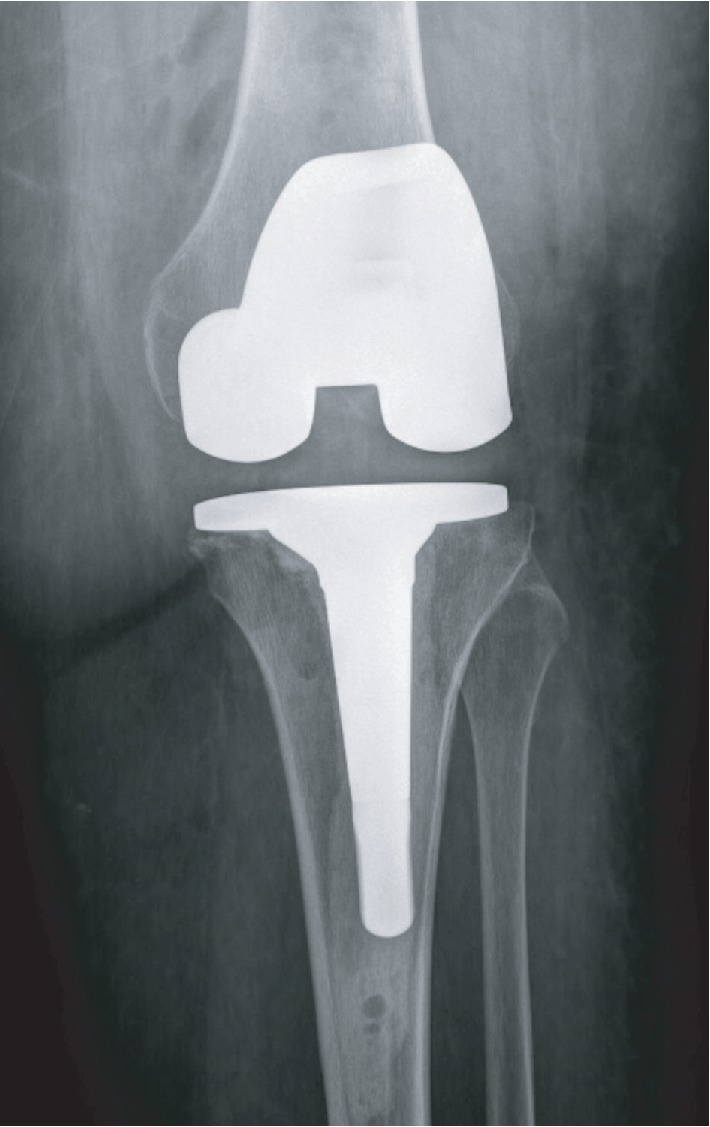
Postoperative AP radiograph of the left knee. Revision left total knee in situ. Pin holes used for navigation are present distal to the stemmed tibial component.
